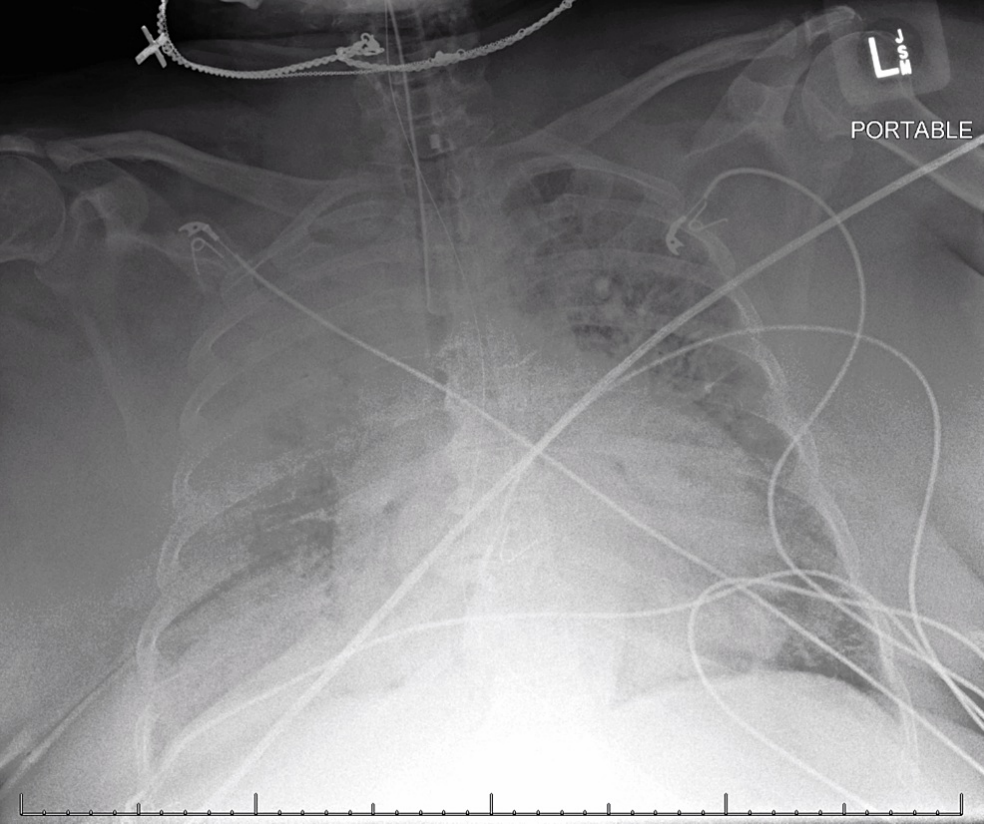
Following intubation, the first portable chest radiograph shows mild cardiomegaly, background interstitial pulmonary edema, dense consolidation in the right upper lobe, and patchy opacity at the right lung base.
